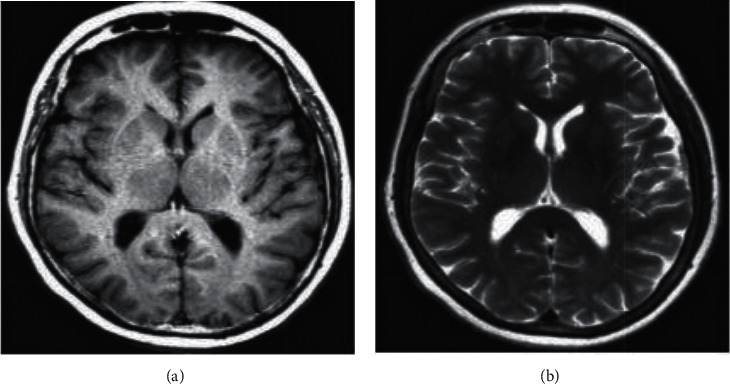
(a) T1-weighted MRI, (b) T2-weighted MRI.
